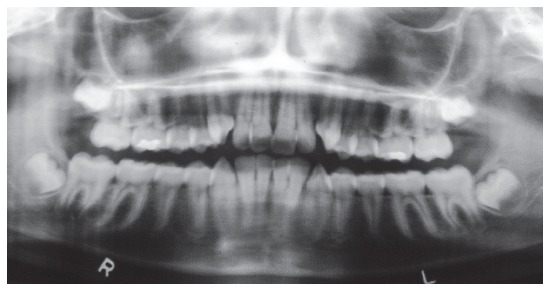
Baseline panoramic radiograph.
